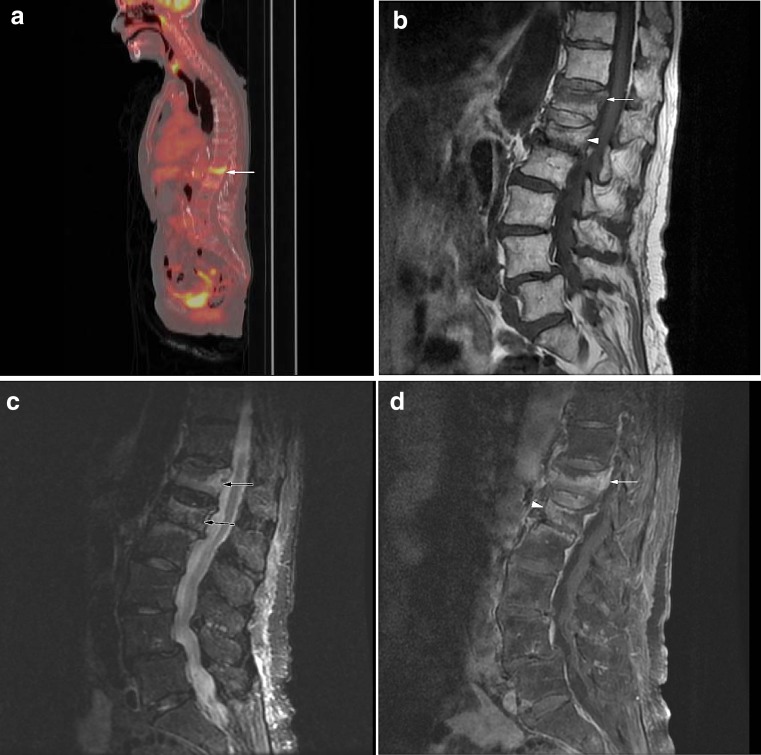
a False-positive compression fracture in a patient with laryngeal cancer. Sagittal fused FDG-PET/CT image demonstrates increased radiotracer uptake in the T12 compression fracture (SUV = 4.9;* arrow*). b Sagittal T1-weighted MRI demonstrates compression fracture of T12 with linear low signal of the superior endplate and preservation of the normal marrow signal (*arrow*). Note compression fracture of L1 with relative preservation of normal marrow signal (*arrowhead*). c Sagittal STIR image demonstrates hyperintensity of the superior endplates of the T12 and L1 compression fractures, suggesting acute fractures (*arrows*). d Sagittal fat-suppressed T1-weighted MRI following the administration of gadolinium demonstrates enhancement of the T12 compression fracture (*arrow*). Mild enhancement is noted, involving the L1 compression fracture (*arrowhead*). Findings were thought to be benign on MRI and on follow-up imaging, there was resolution of bone marrow edema and enhancement
